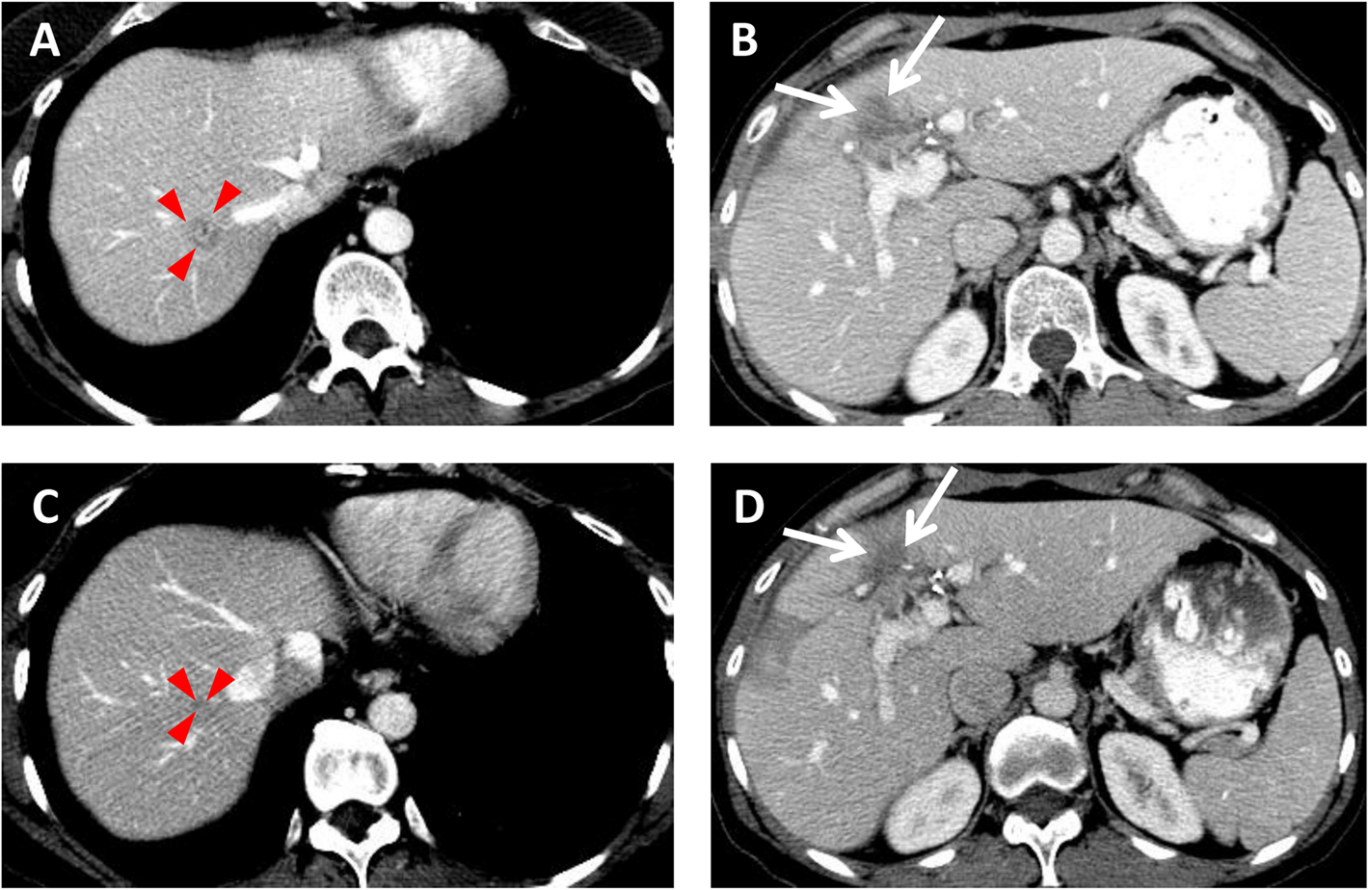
A 45-year-old female with metastatic gallbladder carcinoma. Axial contrast-enhanced CT images demonstrate a a 1.5-cm liver metastasis (*arrowheads*) and b prominent soft tissue (*arrows*) in the cholecystectomy bed abutting the liver. After 2 months of treatment with trastuzumab, c the liver metastasis is barely visible at 4 mm, and d the soft tissue mass in the resection bed, representing recurrent tumor, is decreased. She was stable for an additional 5 months, then had recurrence in the resection bed
